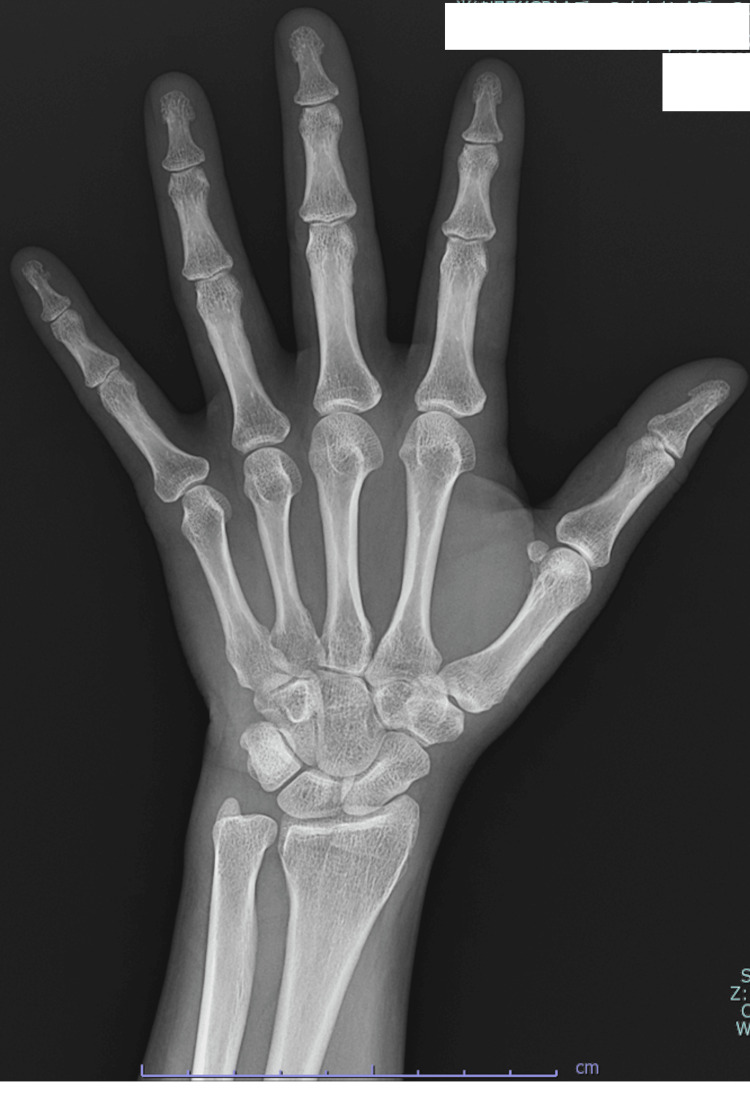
X-ray of the left hand No findings suggestive of a rheumatoid joint were noted.
